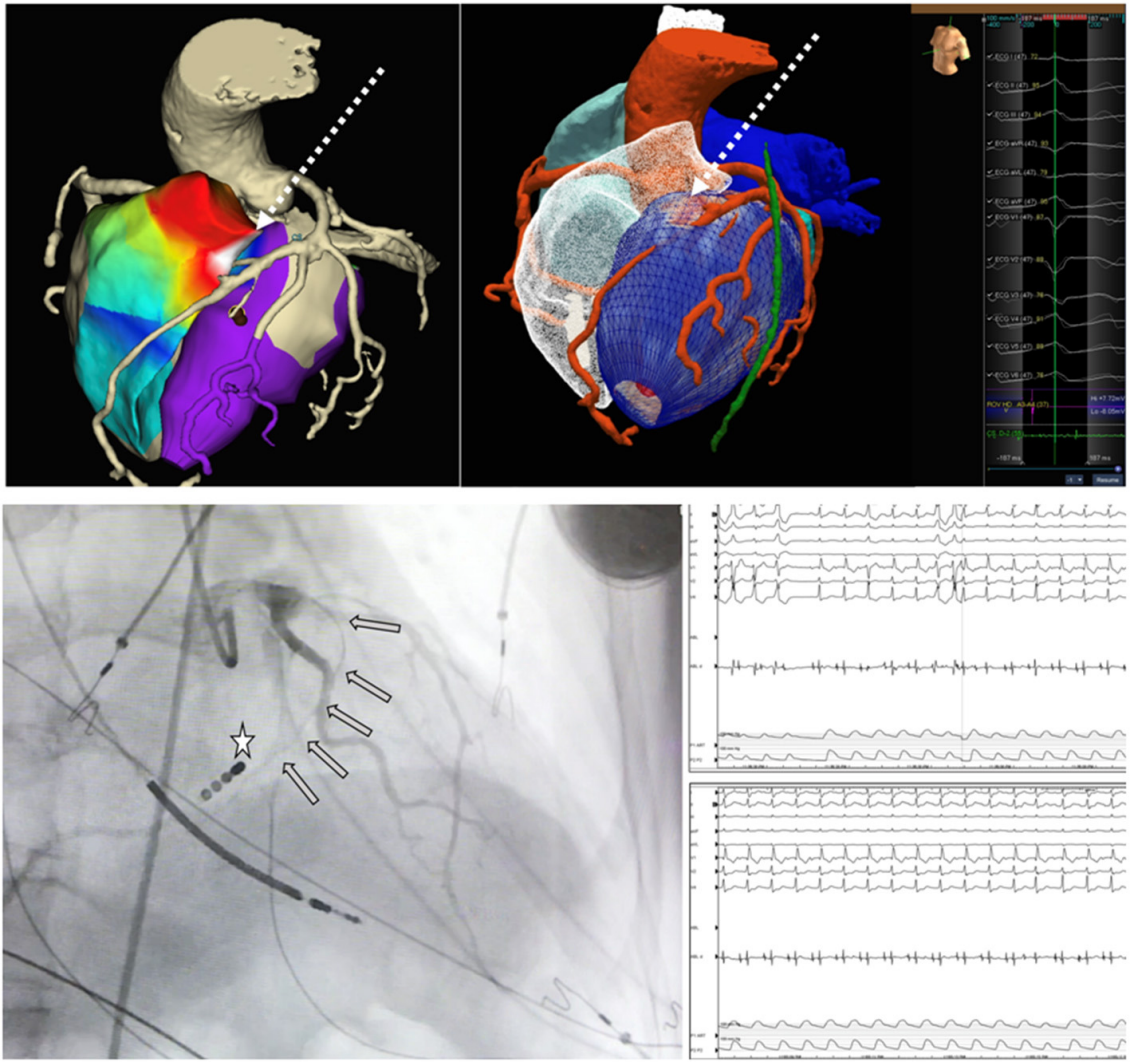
Adapted from Roca-Luque et al. (30). Pace-map electroanatomical map (EAM) of right ventricle (RV) (anterior view) with the highest concordance with ventricular tachycardia morphology (92%) in the anterior RV septum. Ablation as unsuccessful from both right and left ventricle. EAM is merged with pre-procedural cardiac tomography (CT) scan and a septal artery is observed irrigating the area of best pace-map. Centre panel shows only CT scan with septal artery irrigating an area of intramural eptal scar (red) corresponding to the area of best pace-map. Bottom panels show septal alcolholisation of the culprit area according pre-procedural imaging The wire inside septal artery (grey arrows) is shown in front of catheter tip (white star) in the RV septum. After alcholisation at that region (bottom right panel) ventricular tachycardia stops.
